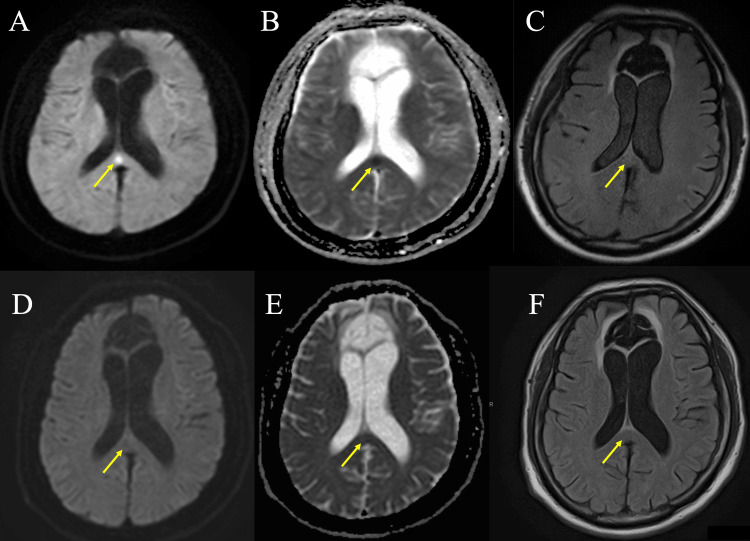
Case 8: MRI findings of CLOCCs (A) DWI shows a high-signal-intensity lesion in the corpus callosum (yellow arrow). Previous bilateral frontal lobe infarcts are also visible. (B) The ADC map shows a corresponding low-signal-intensity lesion, consistent with cytotoxic edema (yellow arrow). (C) FLAIR imaging shows no significant signal abnormality in the corpus callosum (yellow arrow). (D-F) Follow-up MRI shows complete resolution of the corpus callosum lesion on all sequences. ADC, apparent diffusion coefficient; CLOCCs, cytotoxic lesions of the corpus callosum; DWI, diffusion-weighted imaging; FLAIR, fluid-attenuated inversion recovery
